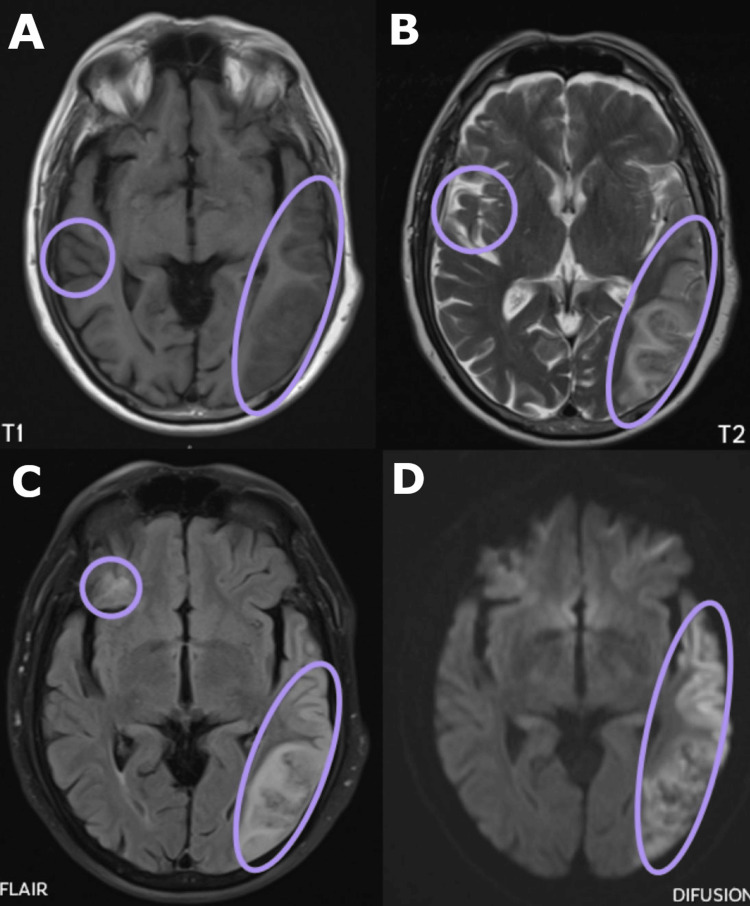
Simple cranial magnetic resonance imaging Panel A (T1 sequence) demonstrates hypointense regions (outlined by purple circles) within the left temporal and right temporoparietal lobes. Panels B (T2 sequence), C (fluid-attenuated inversion recovery (FLAIR) sequence), and D (diffusion sequence) reveal hyperintense areas (highlighted by a purple circles) accompanied by sulcal effacement in the right temporoparietal and left temporal lobes, which are indicative of cerebral ischemia
